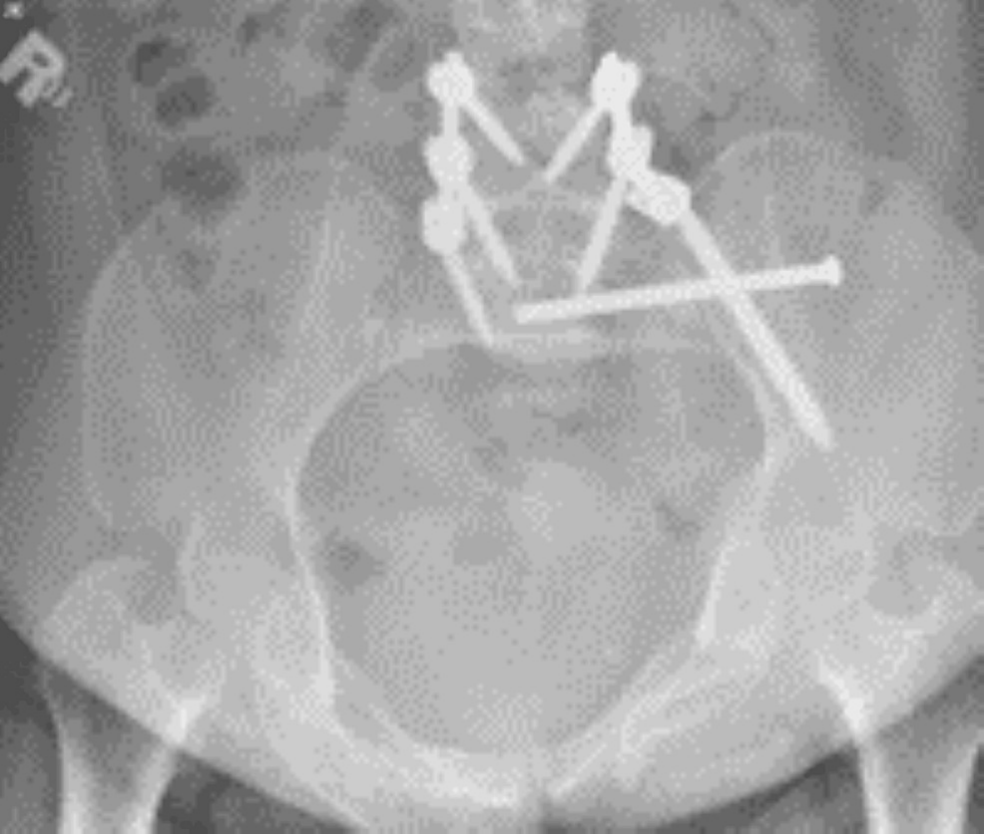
Radiograph demonstrating postoperative in situ spinopelvic fixation percutaneous sacroiliac screw fully threaded at S1 inserted.
